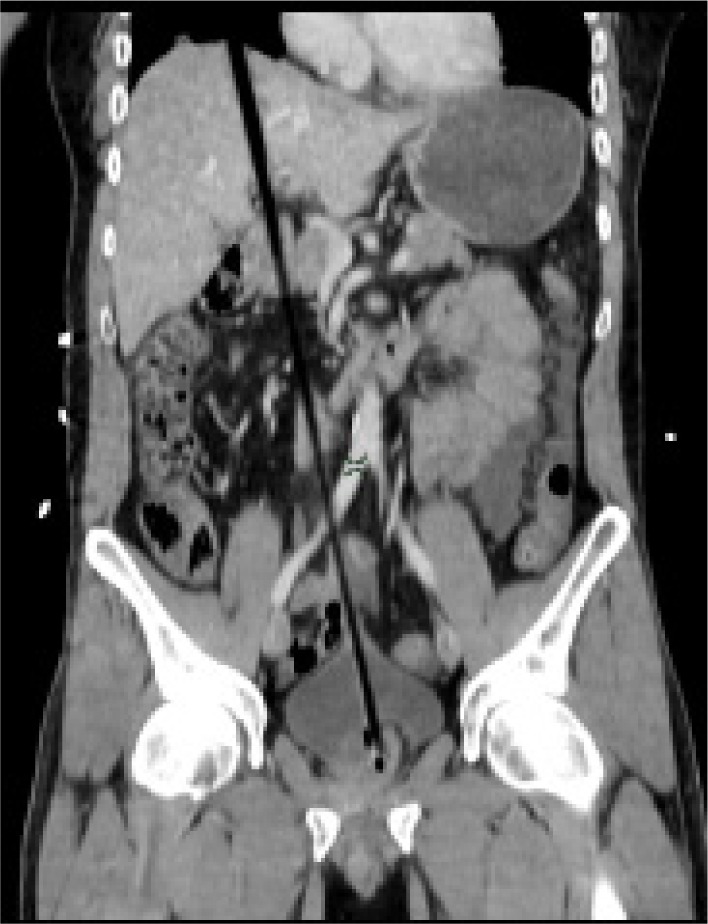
Coronal computed tomography of abdomen and pelvis showing extent of impalement
